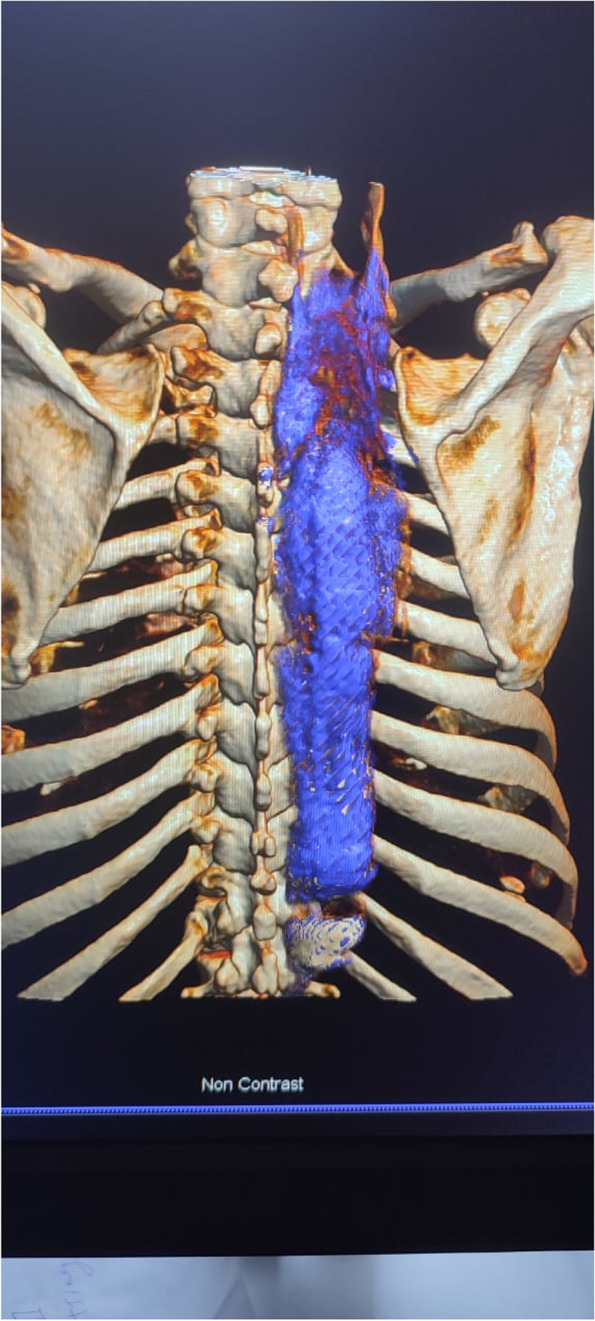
3 D reconstruction by CT (postero-anterior view) of the craniocaudal spread of LA-dye mixture
